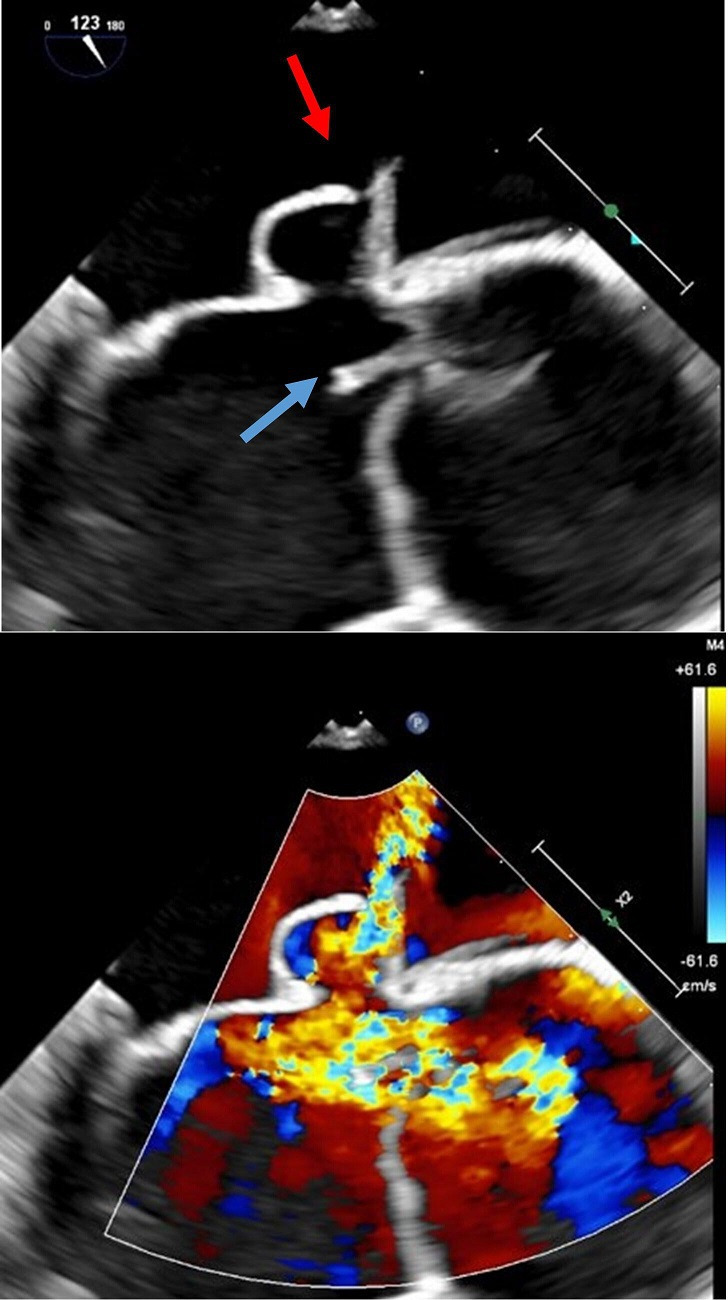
Top: transoesophageal echocardiogram demonstrating valvular vegetation (blue arrow) and mitral valve outpouching and perforation (red arrow). Bottom: the regurgitant jet through the aortic valve perforation (courtesy of Walter Reed Cardiology).
